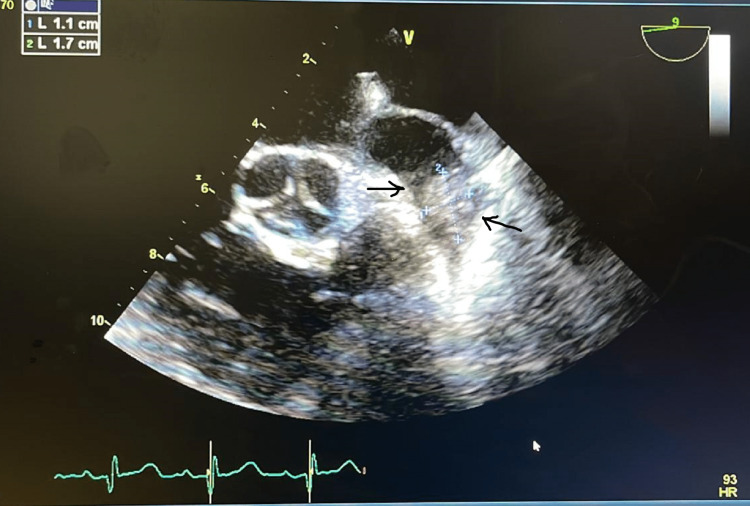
Echocardiogram imaging (apical three-chamber view) showing 3.2x2.0 cm mass in the left atrium (arrows)
